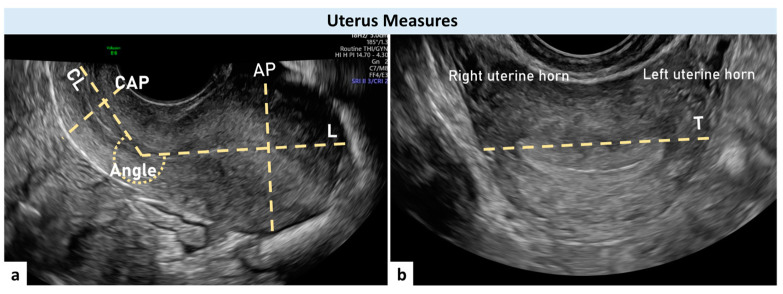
Ultrasonographic image of a retroflexed uterus in a sagittal (a) and transverse scan (b). The interrupted lines indicate uterus measures. L: longitudinal uterus measure; AP: anterior-posterior uterus measure; CAP: cervix antero-posterior diameter; CL: cervix length; ICO: internal cervical os; ECO: external cervical os; Angle: angle of uterine flexion; Probe: ultrasound probe; T: transverse uterus measure.
